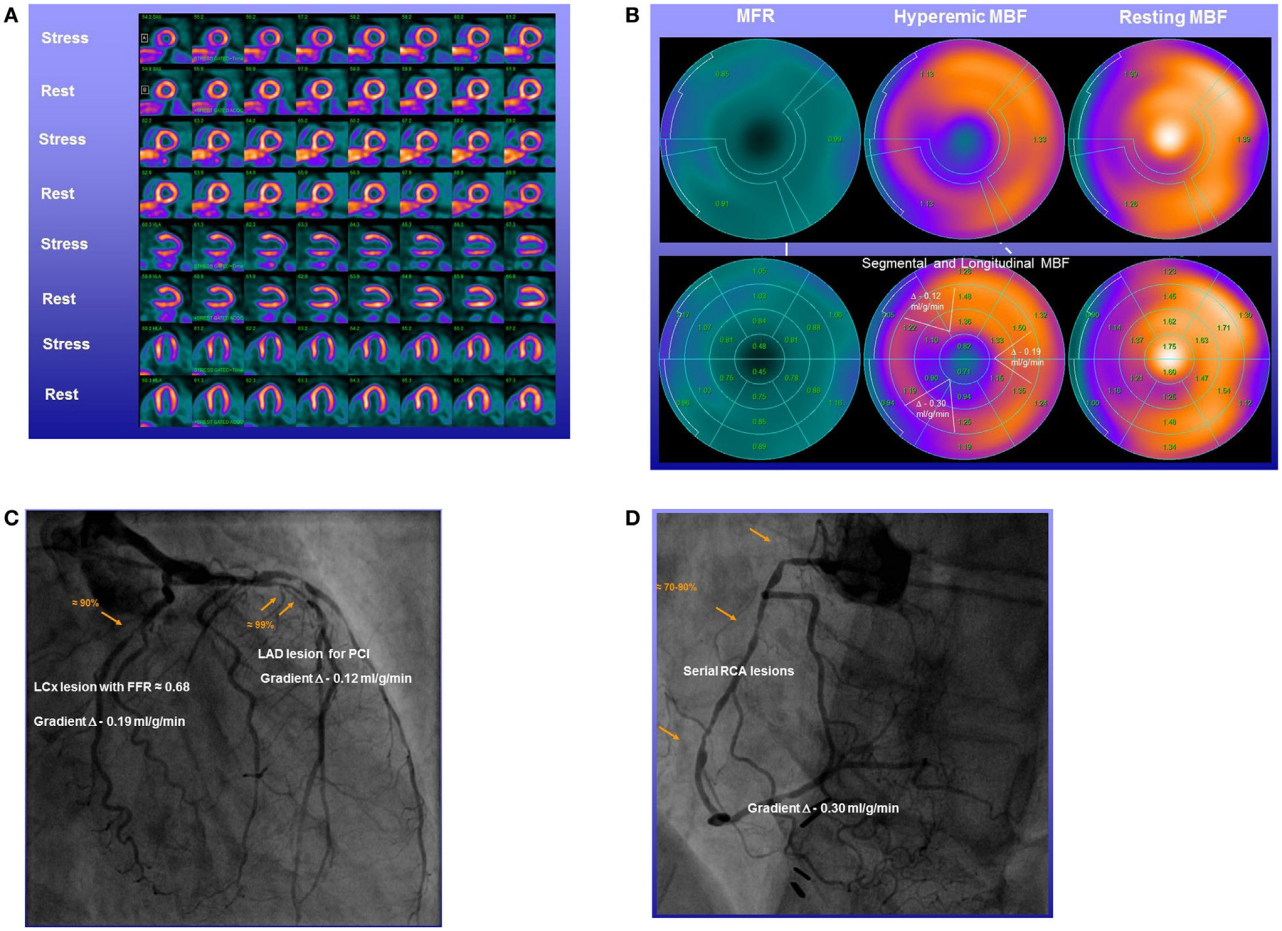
Abnormal longitudinal flow gradient in multivessel CAD. Stress–rest ^13^N-ammonia positron emission tomography/computed tomography (PET/CT) myocardial perfusion and flow quantification with ^13^N-ammonia PET/CT in a 58-year-old man with effort-induced angina chest pain. (A) Regadenoson-stress and rest ^13^N-ammonia PET/CT images in corresponding short-axis (top), vertical long-axis (middle), and horizontal long-axis (bottom) slices. On stress images, a moderate-to-severe decrease in myocardial perfusion, involving the antero-septo-apical and apical wall, is observed that normalizes on rest images to indicate ischemia in the left anterior descending artery (LAD) distribution. (B) Regional myocardial blood flow (MBF) quantification demonstrates abnormally reduced hyperemic MBFs and myocardial flow reserve (MFR) in all three major coronary artery territories of the LAD, left circumflex artery (LCx), and right coronary artery (RCA), respectively (upper panel). Segment MBF analysis unmasks a decrease in MBF from the mid to distal segments with a mean longitudinal MBF gradient during hyperemic flow in the LAD (0.12 ml/g/min), LCx (0.19 ml/g/min), and RCA (0.30 ml/g/min) (lower-middle panel). (C) Invasive coronary angiography of the left coronary artery in this patient showed a long 99% stenosis in the mid-LAD that was responsible for the observed stress-induced perfusion defect of the antero-septo-apical and apical walls on ^13^N-ammonia PET/CT perfusion images. In addition, large caliber diagonal branches of the LAD present a ≈70–80 and ≈80–90% stenosis, respectively. The proximal LCx has a proximal ≈80–90% stenosis. Invasively measured fractional flow reserve (FFR) of the proximal LCx lesion was abnormally reduced with 0.68 emphasizing also the hemodynamic significance of this lesion. (D) Invasive coronary angiography of the RCA demonstrates serial epicardial lesion from the proximal to mid segments of ≈70–90%, respectively [adapted with kind permission of Valenta et al. (26)].
